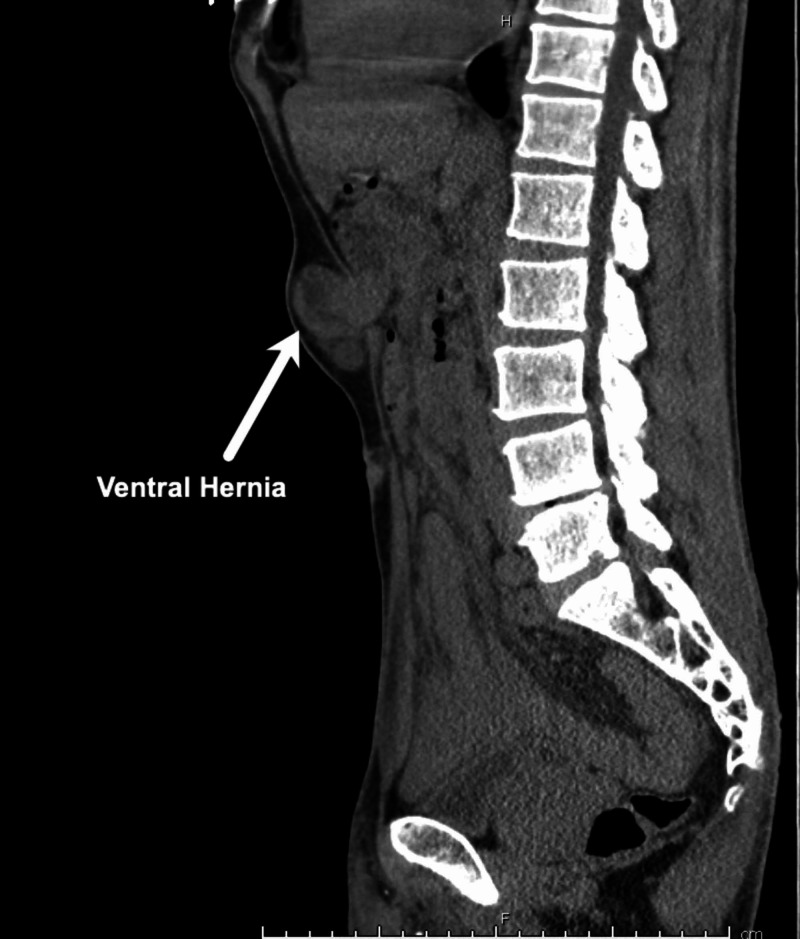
Case 2: Sagittal CT scan.
